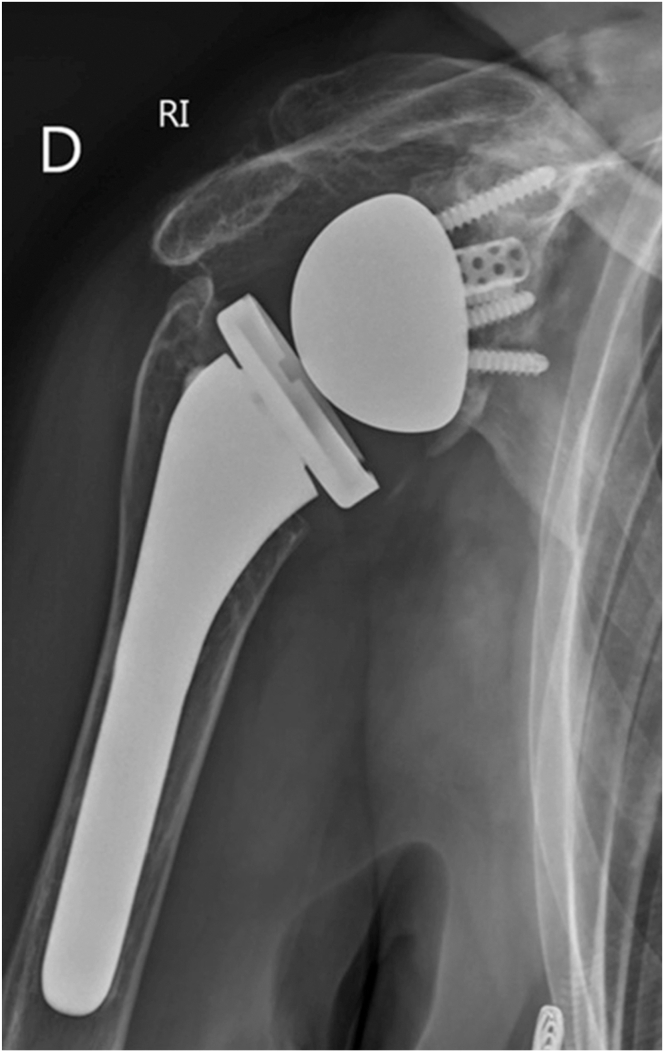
Right anteroposterior x-ray of a reverse shoulder arthroplasty complicated by a scapular spine fracture. The inferior tilt has led to secondary impingement.
